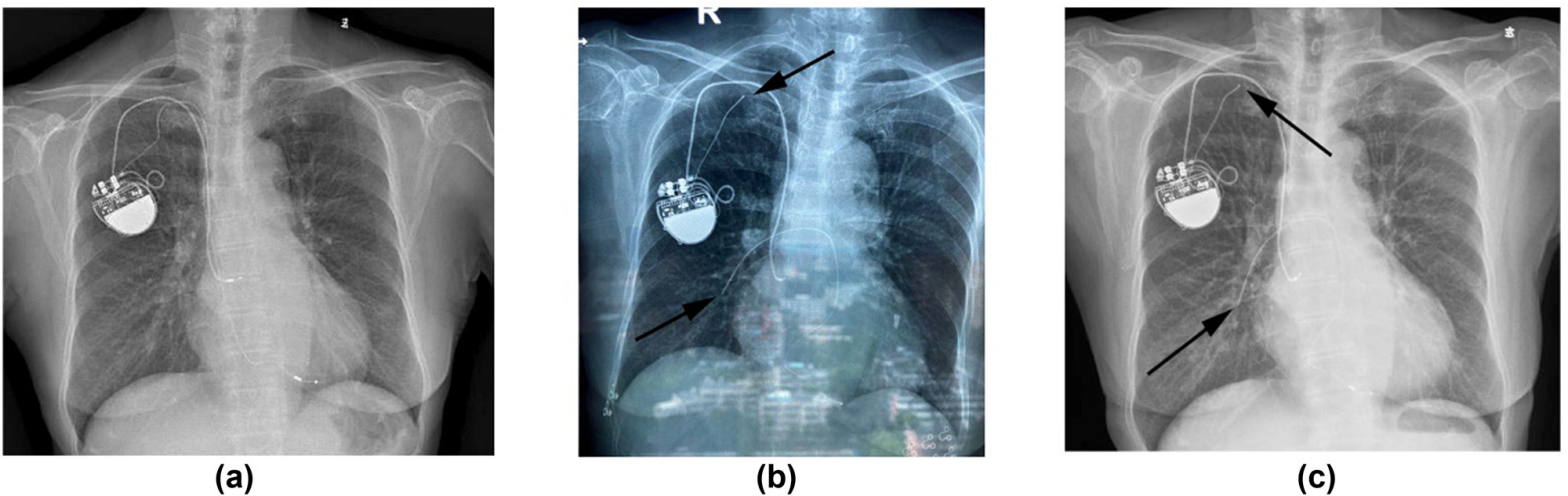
Chest X-ray images from December 2019 (a) showing both leads were intact, while results from January 2020 (b) and September 2020 (c) showing a complete right-ventricular lead fracture (arrow).
